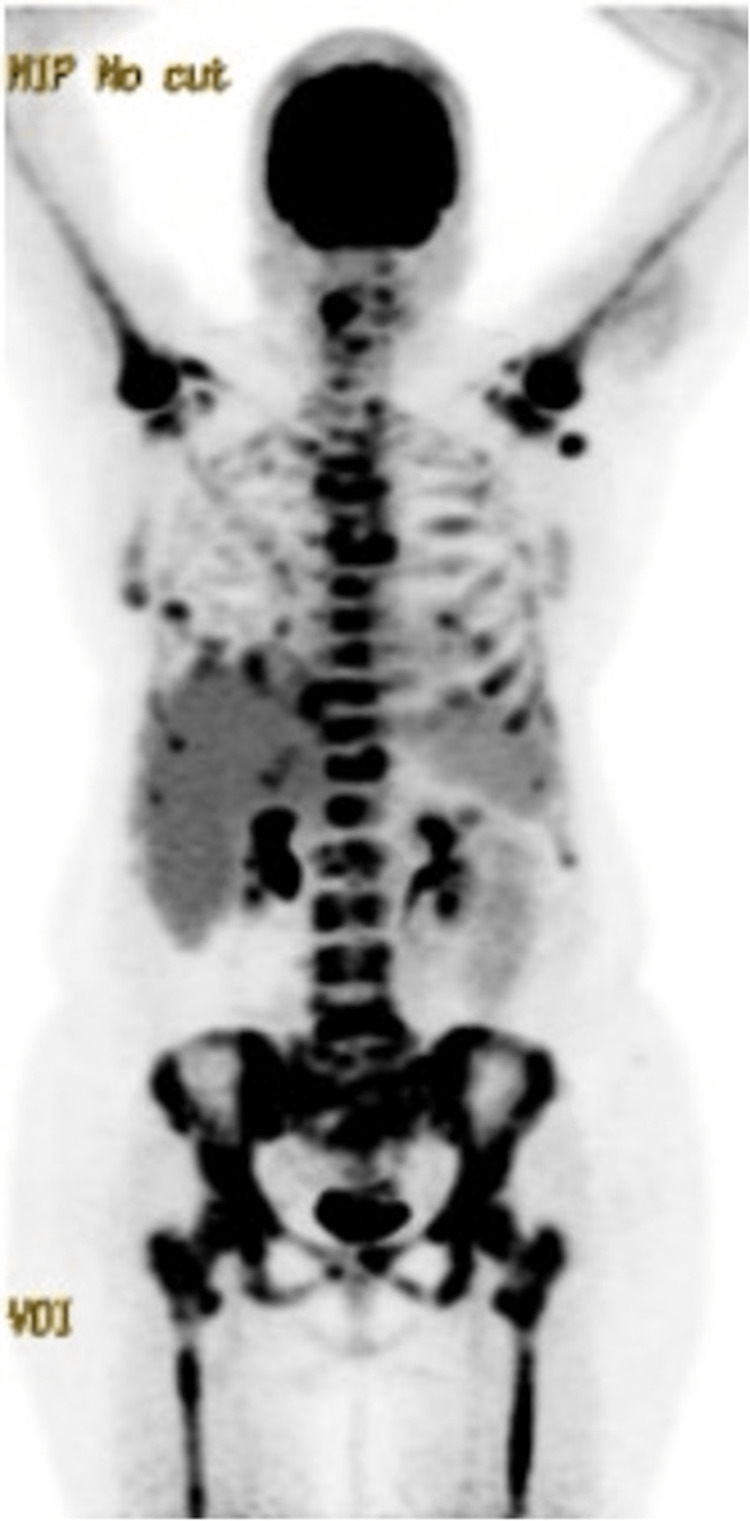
FDG-PET/CT scan showing diffuse osseous involvement of the appendicular and axial skeleton, left axillary lymph node involvement, bilateral hilar lymphadenopathy, bilateral pulmonary spiculated nodules, abdominal lymph nodes (mesenteric, retroperitoneal, and left common iliac chain) involvement, and liver involvement FDG-PET: fluorodeoxyglucose-positron emission tomography
